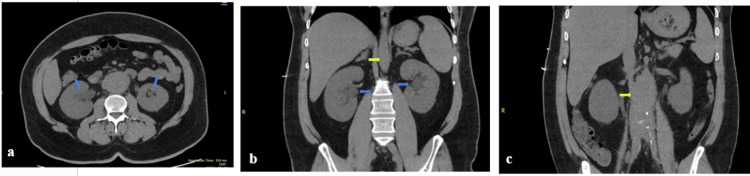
CT KUB demonstrating retroperitoneal fibrosis with bilateral hydronephrosis. Axial (a) and coronal (b and c) non-contrast CT KUB images demonstrating bilateral hydroureteronephrosis (blue arrows) with an associated lobulated retroperitoneal soft-tissue mass encasing the abdominal aorta, inferior vena cava, and bilateral ureters (yellow arrows), consistent with retroperitoneal fibrosis. CT KUB: Computed tomography of kidneys, ureters and bladder
